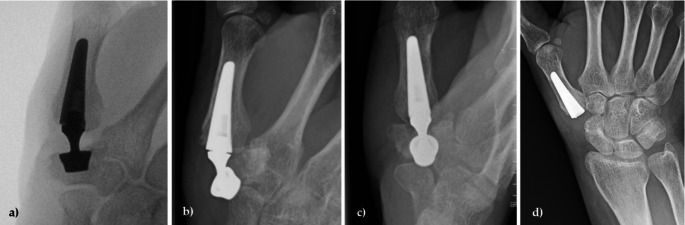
Radiographs of a patient before and after revision surgery. a) Intraoperative radiograph during initial surgery; b) and c) Cup dislocation 7 weeks after primary surgery due to fracture of the trapezium; d) Revision surgery performed as secondary trapeziectomy
